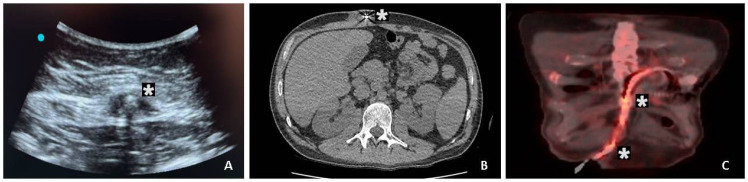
(A) Ultrasonography, (B) computed tomography, and (C) positron emission computed tomography images of patient who was diagnosed with driveline infection. Asterisk points out the infected region.
